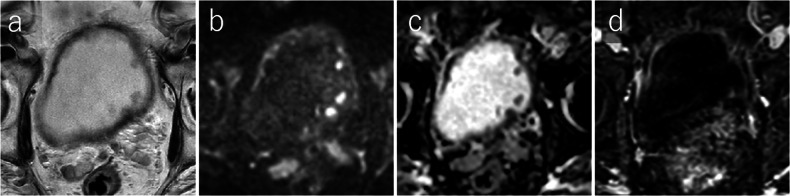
Post-BCG therapy: an 81-year-old man with multiple papillary nodules and broad wall thickening post-BCG therapy MRI shows intermediate signal intensity papillary nodules with broad wall thickening on T2WI (a), high signal intensity on DW-MRI (b), intermediate signal intensity on the ADC map with a mean ADC value of 1.272 × 10^−3^ mm^2^/s (c), and DCE-MRI shows multiple contrast-enhanced nodules (d). ADC, apparent diffusion coefficient; DW, diffusion-weighted; T2WI, T2-weighted imaging; VI-RADS, vesical imaging-reporting and data system
